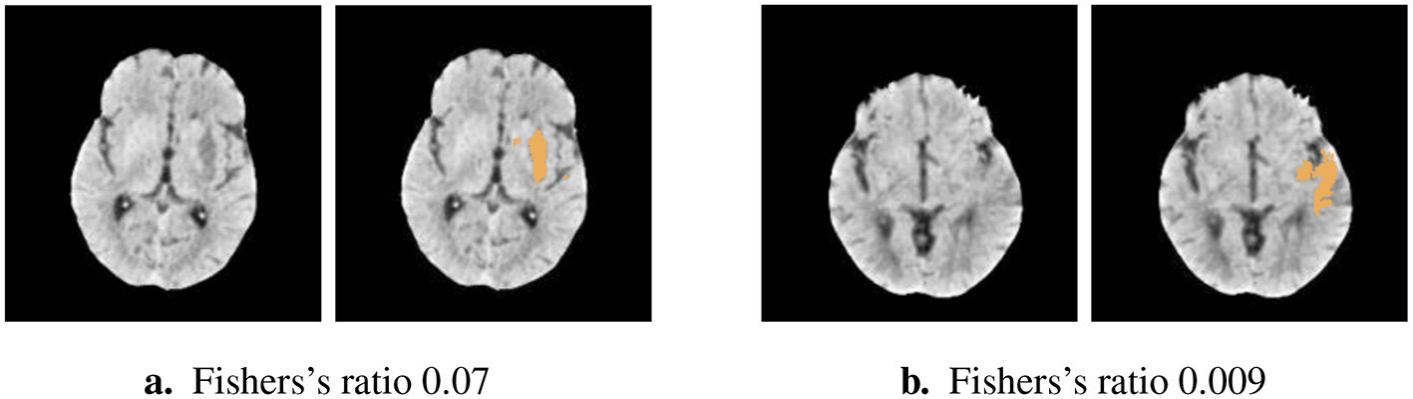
Examples of slices of different lesion contrast with their reference (A) higher contrast (B) lower contrast showing the variability among the dataset and the difficulty of segmentation of stroke lesion segmentation on CT scans.
